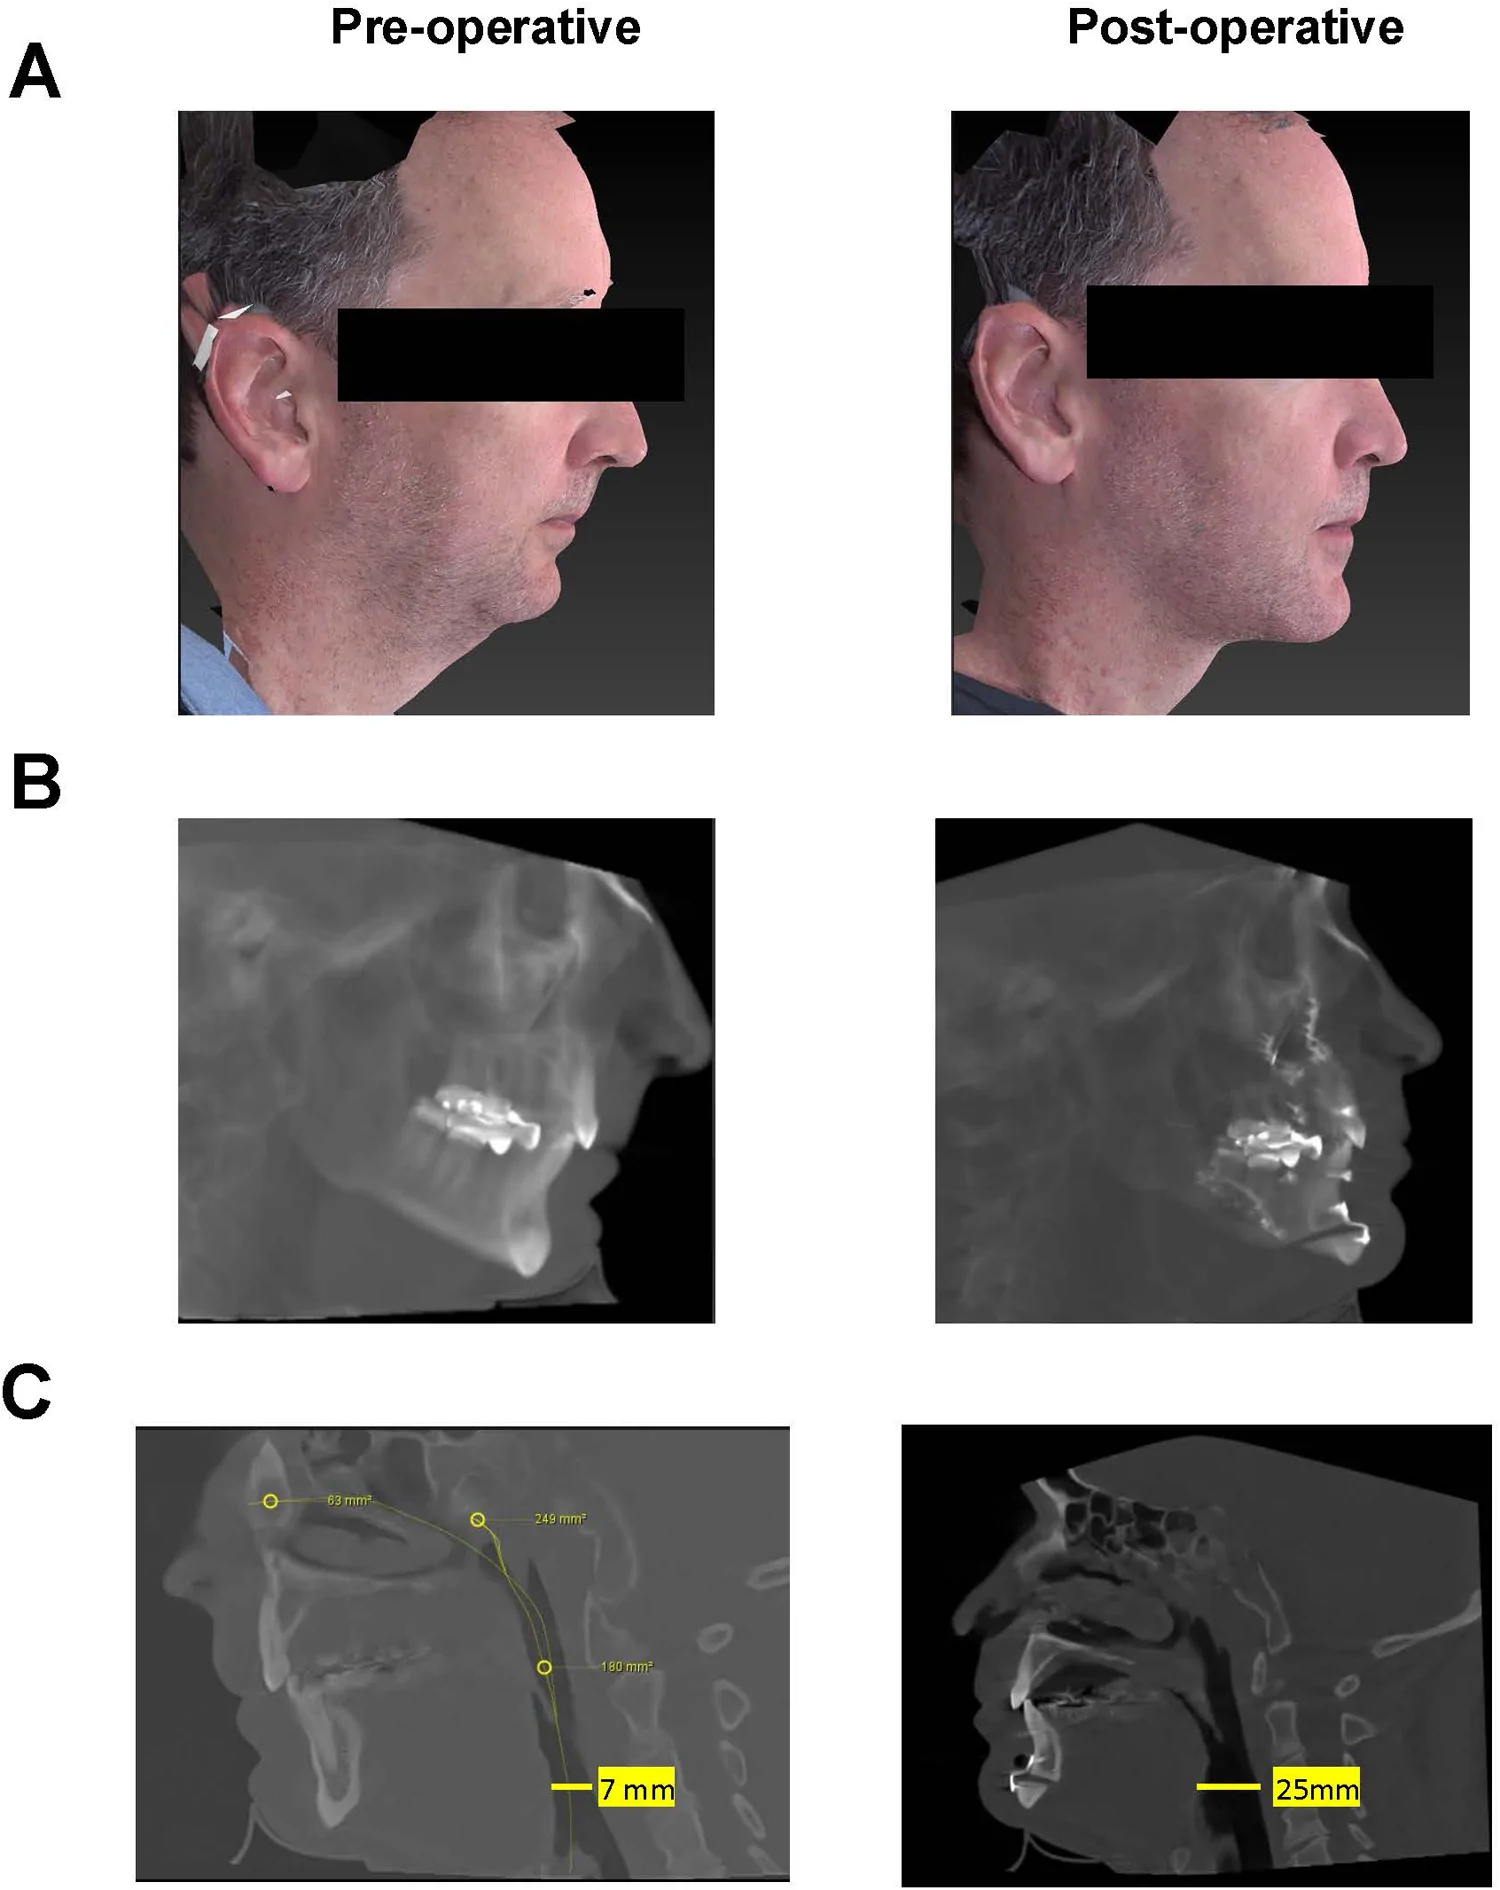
Panel A shows side profile photographs of the same individual before and after MMA, with eyes blacked out for privacy. Panel B contains corresponding pre-operative and post-operative lateral radiographic scans, highlighting skeletal changes. Panel C displays sagittal CT images, with measurements indicating linear airway space increasing from 7 millimeters pre-operatively to 25 millimeters post-operatively.
